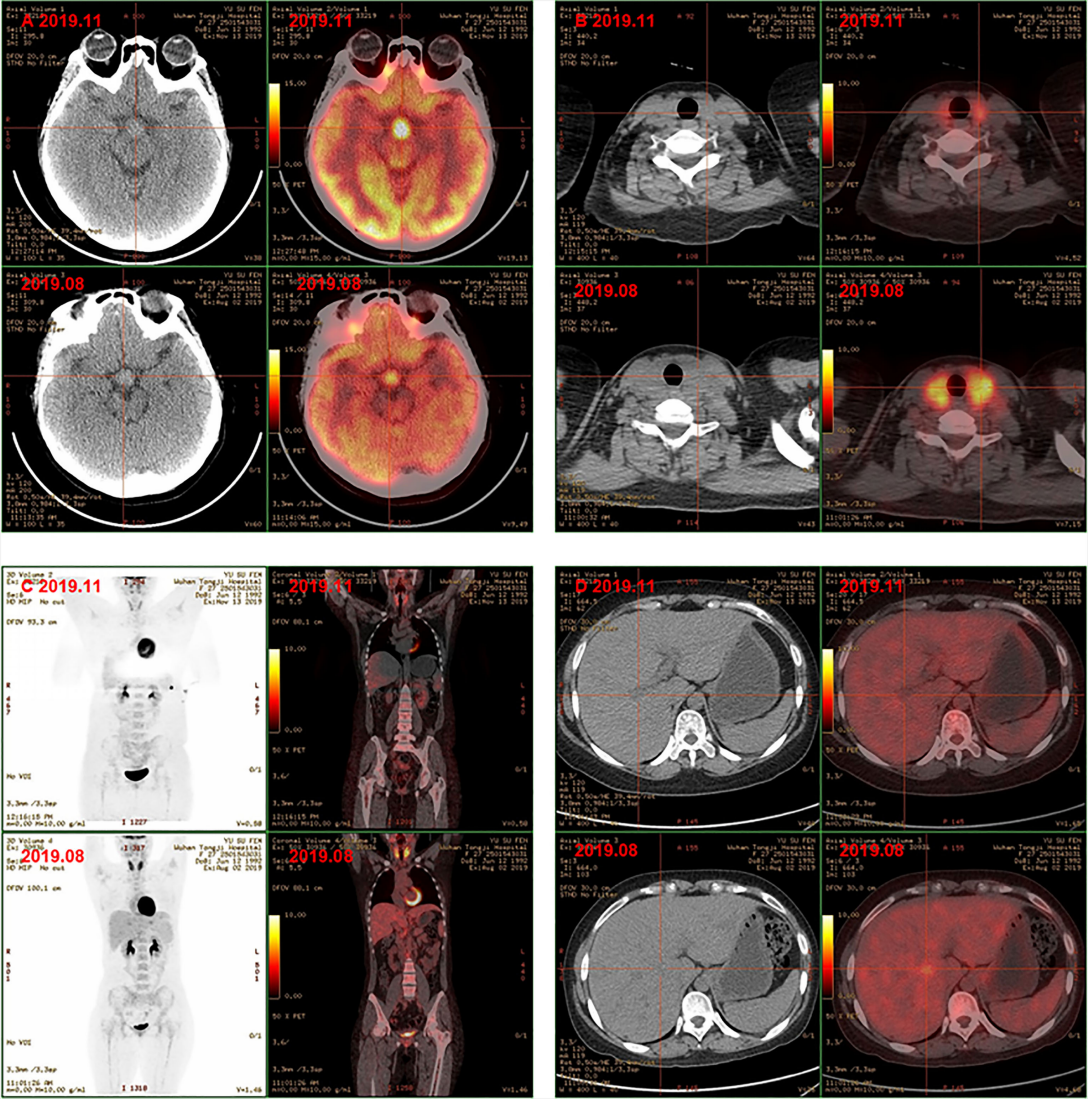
PET-CT showed hyphothalamic nodule was thickened and the metabolism of hypothalamus was higher (A); the volume and metabolism of thyroid gland decreased (B); the range of primary lesions and the metabolism was significantly reduced, the metabolism of bilateral tonsils and bone marrow was similar to the previoud (C); metabolism of the multiple foci in the liver subsided (D) after chemotherapy.
